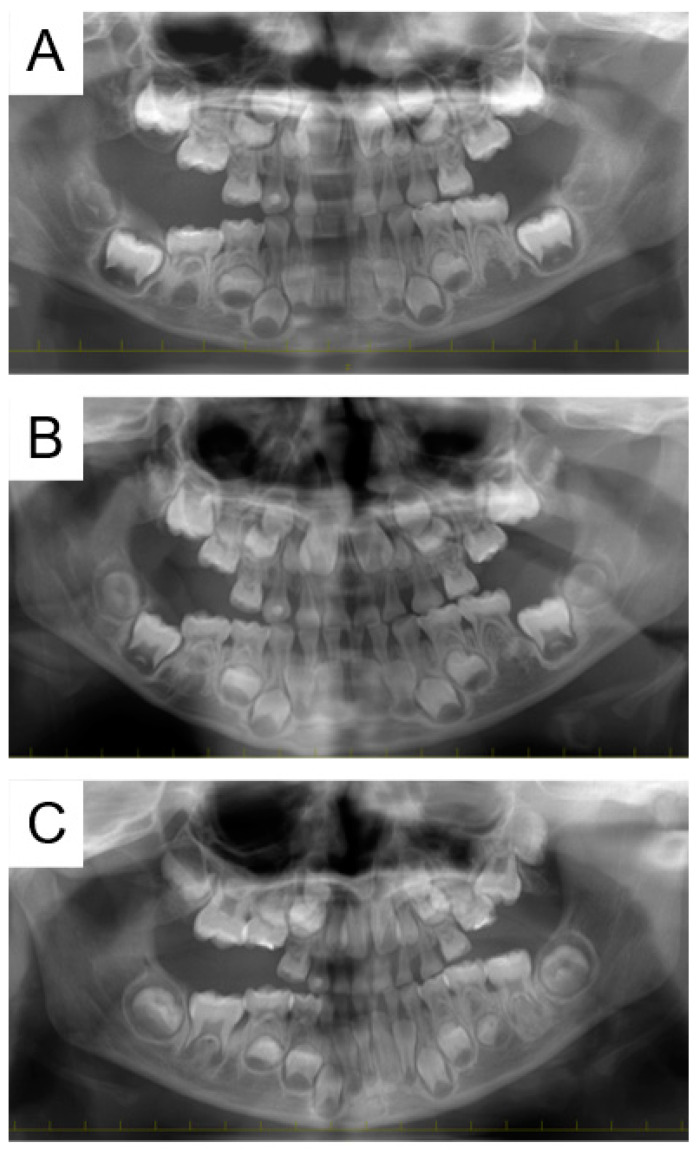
Panoramic radiographs of Case 1 revealing the diagnosis as delayed eruption of the maxillary primary second molars, and the location was not changed despite traction treatment. Images taken at (A) 4 years and 6 months, (B) 5 years and 3 months, and (C) 6 years and 6 months.
